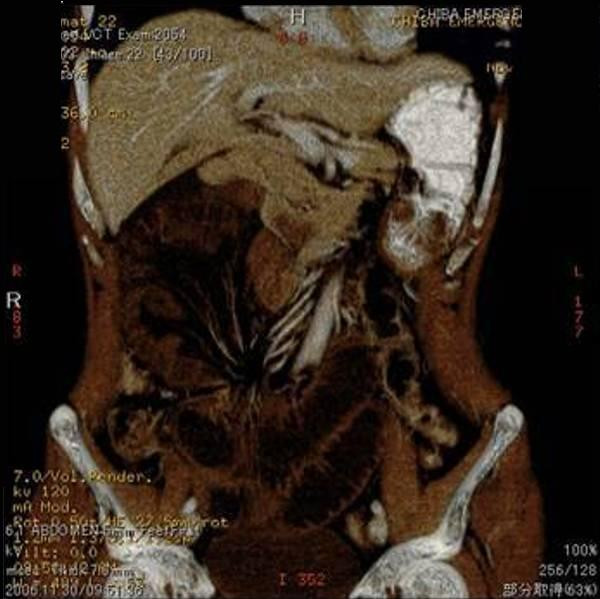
Retrospective multiplanar reconstructed enhanced multidetector-row computed tomography images reveal an abnormal cluster of digestive loops in the right lateral abdominal cavity and mesenteric vessel changes such as twisting and stretching.
